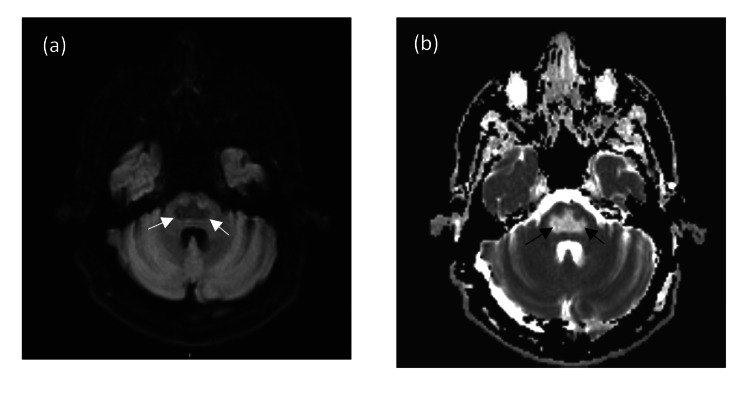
MRI brain: axial DWI (a) and ADC (b) demonstrates T2 shine through with low signal on DWI (between white arrows) and corresponding high signal on ADC map (between black arrows) DWI: diffusion-weighted imaging; ADC: apparent diffusion coefficient
